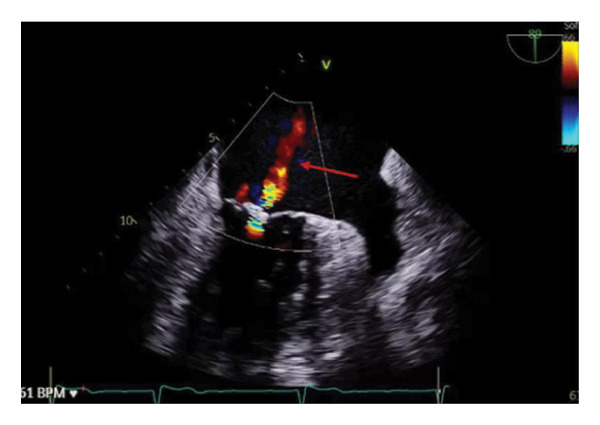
Transesophageal echocardiogram. Mid‐esophageal long axis view. Note the eccentric jet of mitral regurgitation on Doppler directed posteriorly (red arrow). Again seen in the thickened mitral valve and enlarged left atrium.
